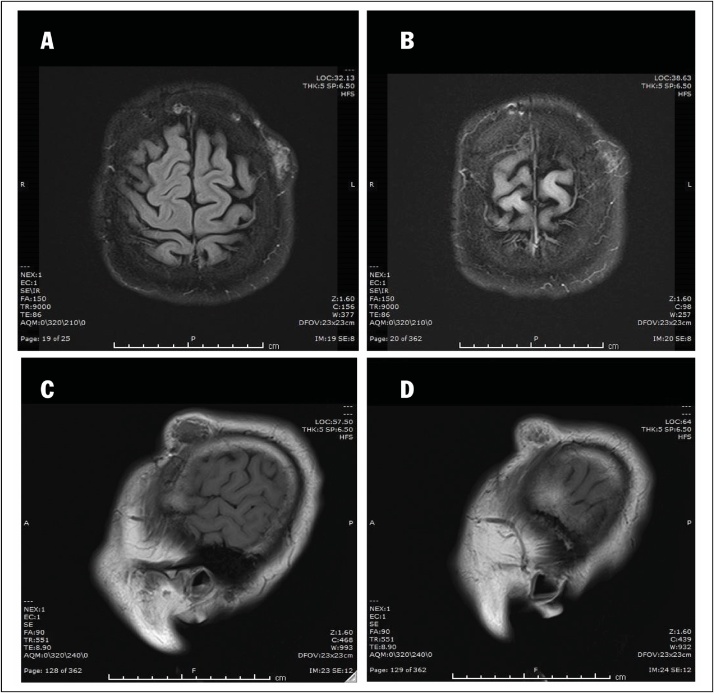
A, B. Post-contrast axial T1-weighted sequence showed a slight homogenous enhancement without any bony invasion. C, D. Post-contrast sagittal T1-weighted sequence showed a homogenous enhance of the left frontoparietal scalp mass, separated from the calvarium.
